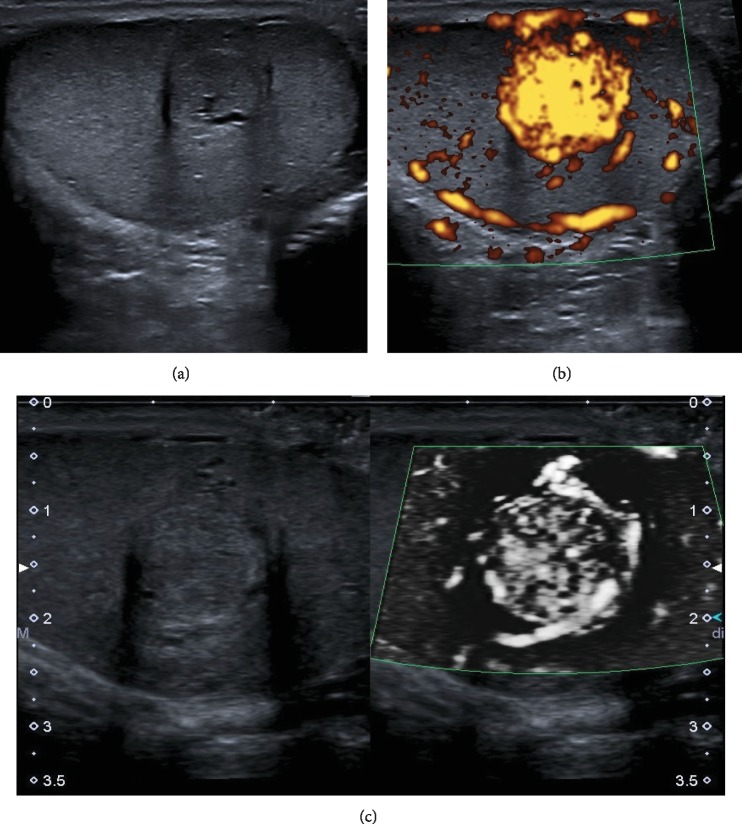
B-mode US, power Doppler imaging, and SMI of a capillary hemangioma. (a) Isoechogenic lesion in the central part of the left testicle with peripheral hypoechogenic rim (11 mm) and central feeder vessel. (b) Power Doppler imaging showed strong vascularization of the lesion compared to the surrounding tissue. (c) Monochromatic SMI in split screen mode determined stronger feeder vessels in the peripheral part of the lesion with high central vascular density. Abbreviations: US: ultrasound; SMI: superb microvascular imaging.
